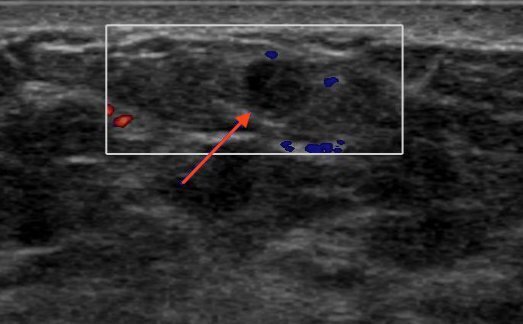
Breast ultrasound confirming the presence of a hypoechoic, well-circumscribed mass on the right side (arrow), with minimal Doppler flow.
